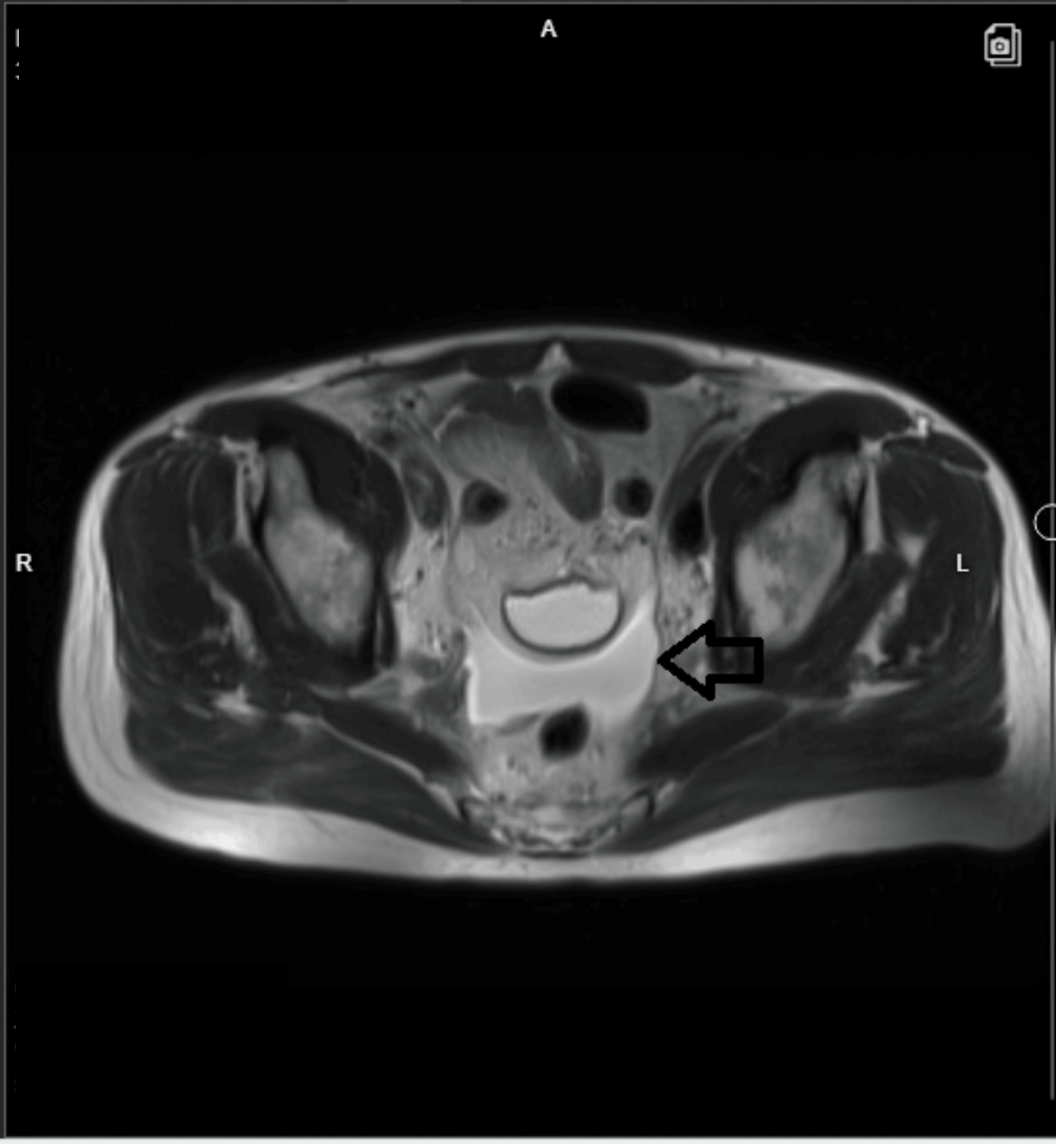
MRI of the abdomen and pelvis (T2-weighted axial view) MRI: magnetic resonance imaging
